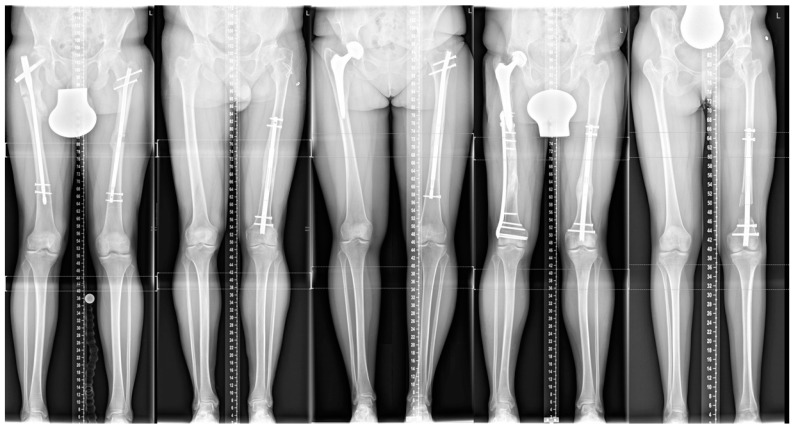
Long-standing radiographs are presenting all five patients (1 to 5).
